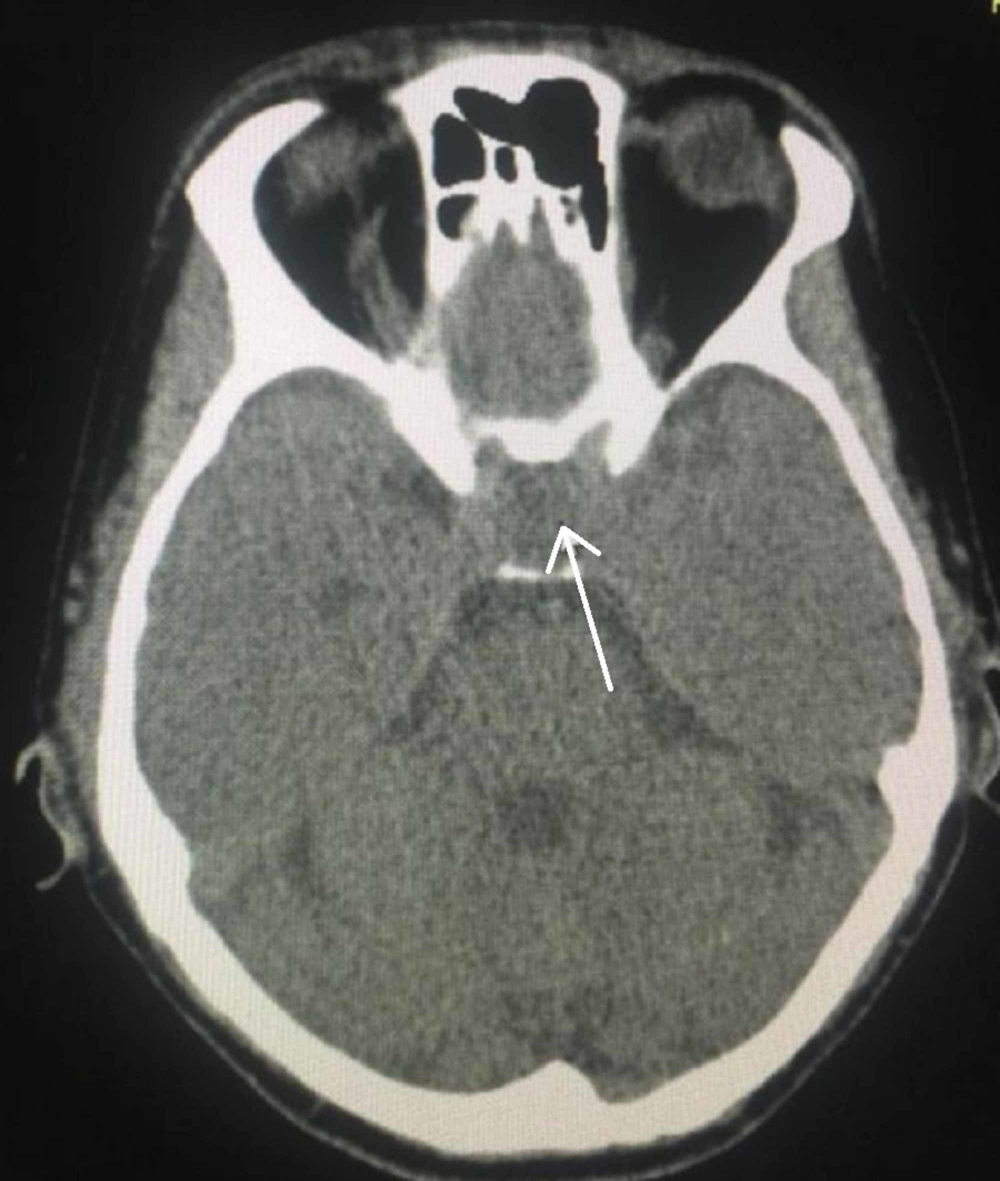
Computed tomography brain showing an iso to hypodense lesion in the sella (arrowhead), causing sellar widening, suggestive of a pituitary lesion, likely a pituitary macroadenoma
